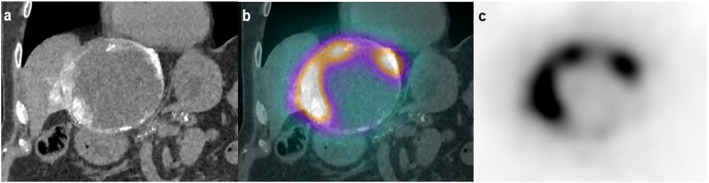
Voluminous HCC tumor with extensive necrosis and viable peripheral tumor in an 81-year-old patient treated with TARE: perfused volume of 81 cm^3^ estimated on pre-treatment ^99m^Tc-MAA SPECT/CT and a subsequent ^90^Y-Res of 11.6% on ^90^Y-PET/CT acquisition post-TARE; a coronal image of intra-arterial CT during TARE with soluble contrast media injected through the microcatheter, b coronal image of fused Bremsstrahlung ^90^Y-SPECT/ CT with intra-arterial CT during TARE and c coronal image of Bremsstrahlung ^90^Y-SPECT
